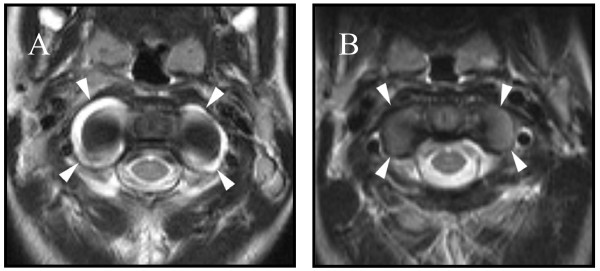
Image A is a T2-weighted proton density axial MR image through the C1–C2 articulations showing effusions (arrows) surrounding the joint spaces. Follow up T2 MR image 4 weeks later (image B) is normal.
